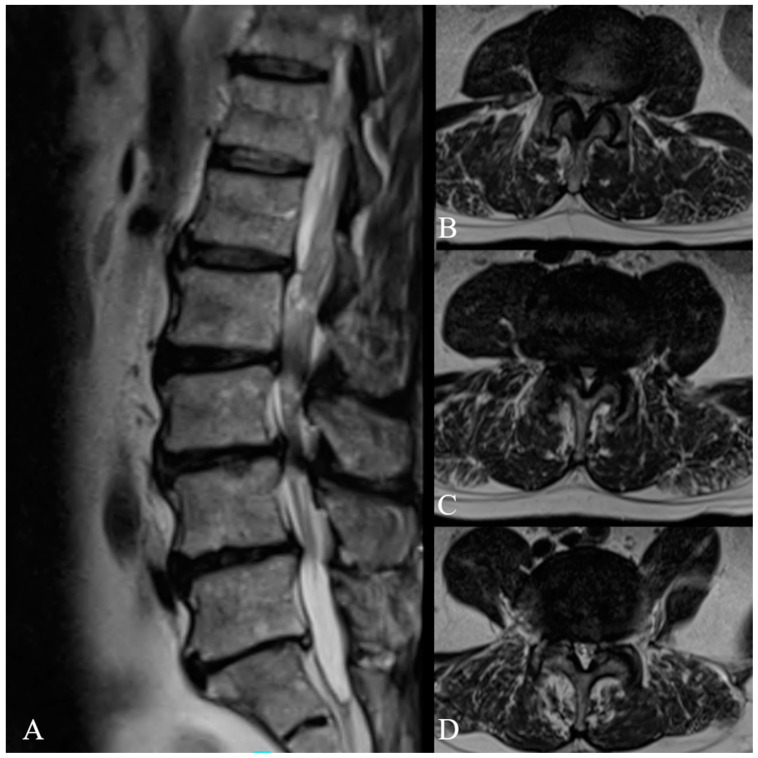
Preoperative MRI demonstrating: (A) multilevel central stenosis, (B) central and bilateral foraminal stenosis at L2-3, (C) central and bilateral foraminal stenosis at L3-4, (D) central and bilateral foraminal stenosis at L4-5.
